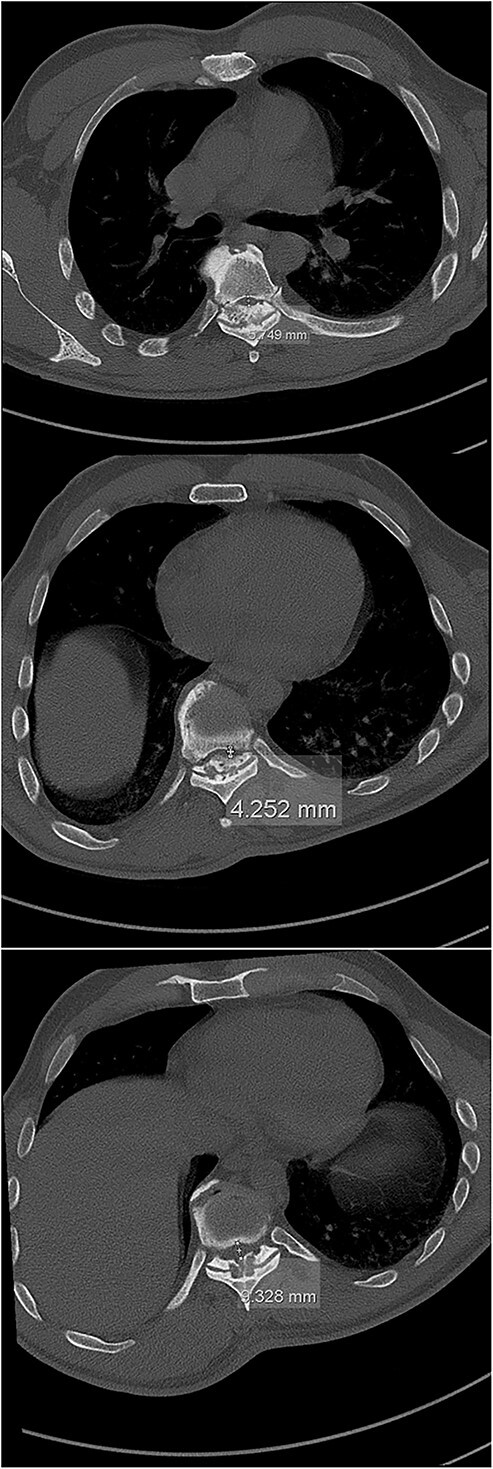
The initial CT scan (axial views), showing the width of the spinal canal at Th6 (above), Th9 (middle) and Th11 (below).
